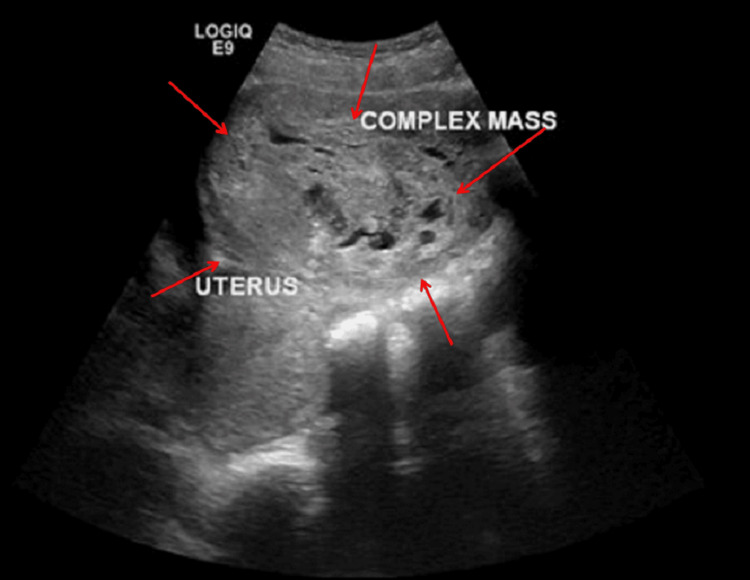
Ultrasound image of uterine mass adjacent to the uterus (Case 2)
